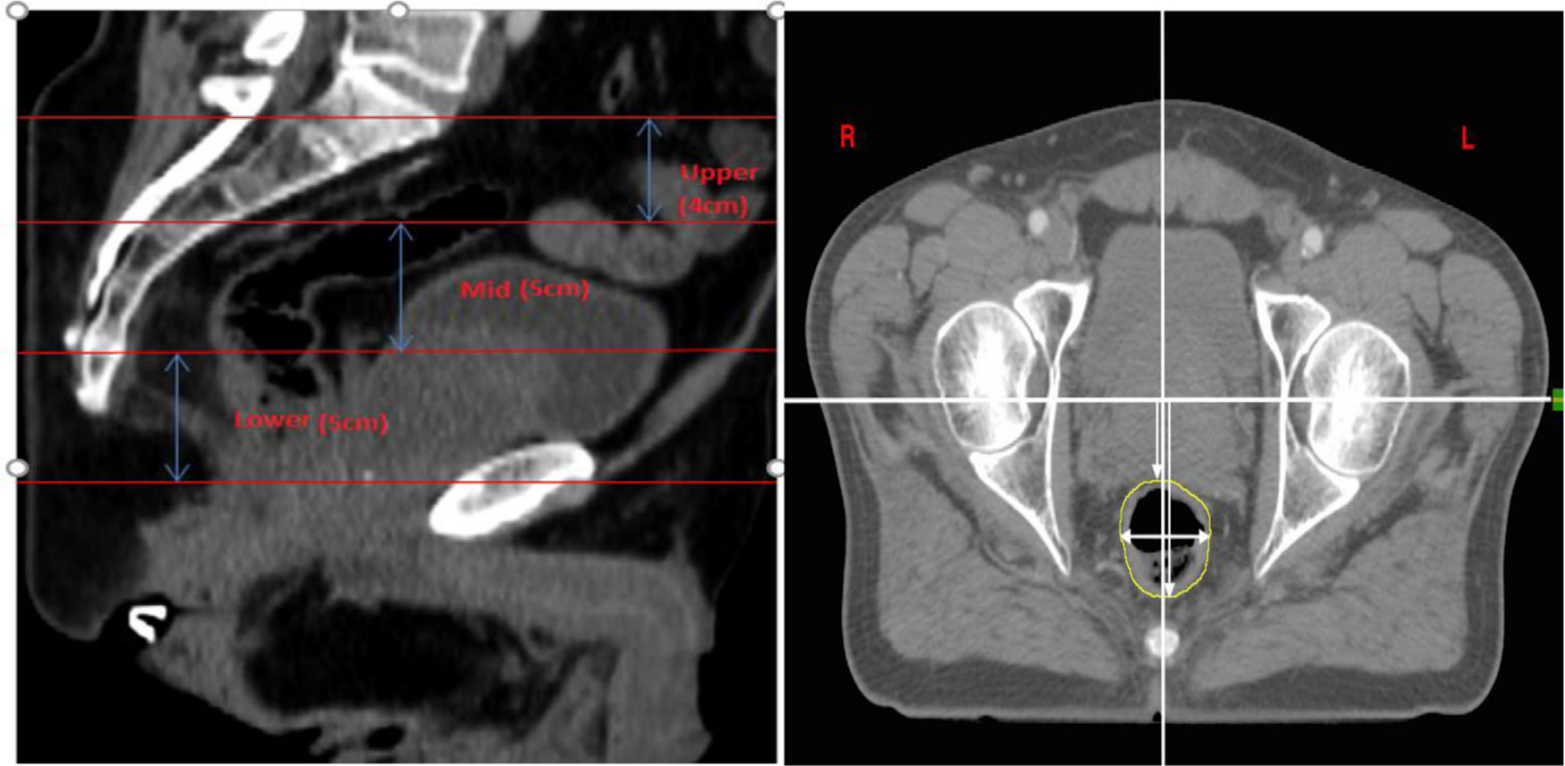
Left: Computed tomography (CT) image showing the upper, mid, and lower rectum as defined to be starting from the superior anterior border of S1. Right: Measurement of anterior, posterior, and lateral rectal wall motion. Anterior and posterior rectal movement was taken from the center of the left femoral head. Lateral rectal movement was taken from the center of the spine.
